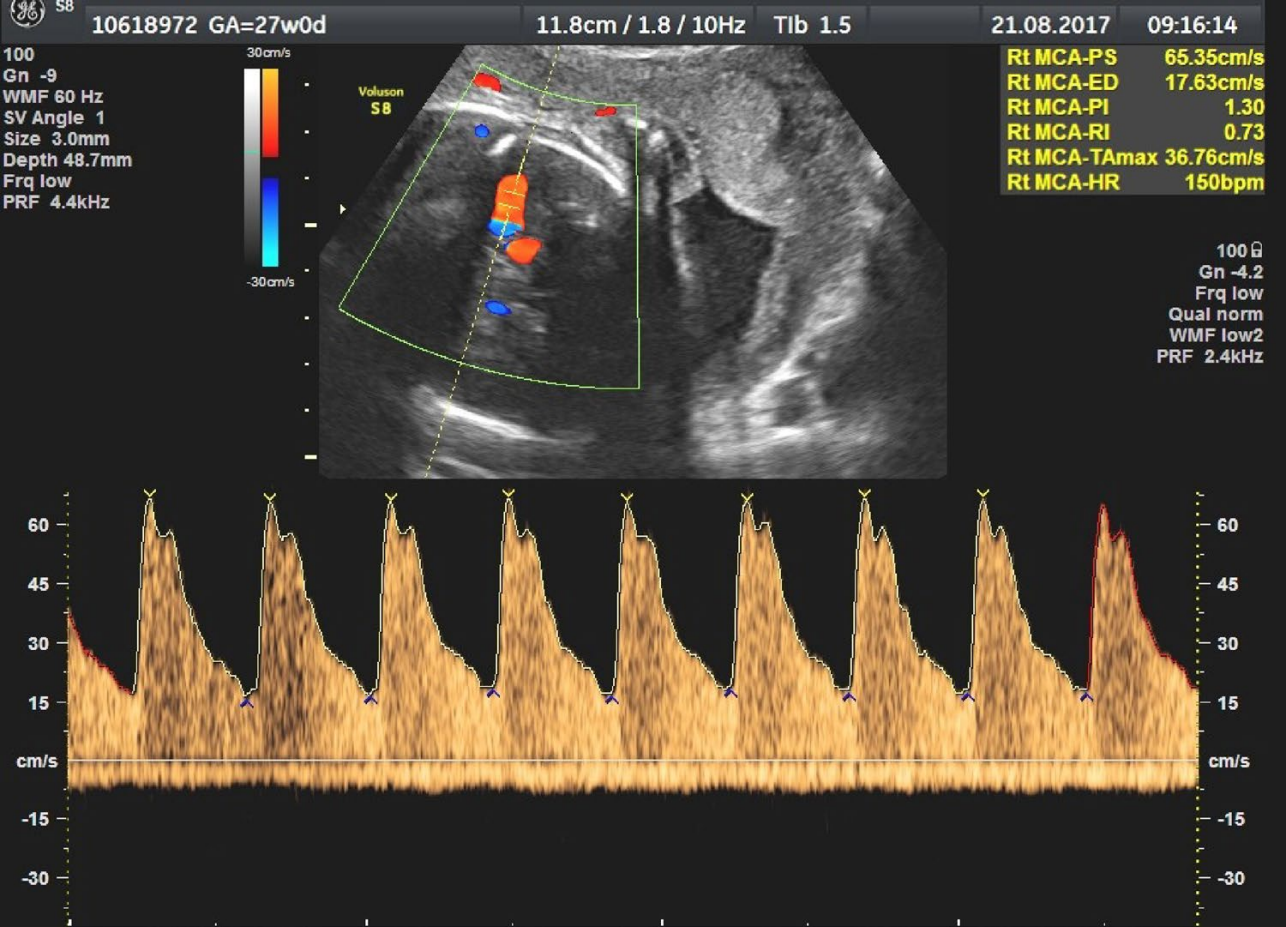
Example of fetal middle cerebral artery (MCA) Doppler with a good image quality presenting a second systolic peak (MCA-P2)
